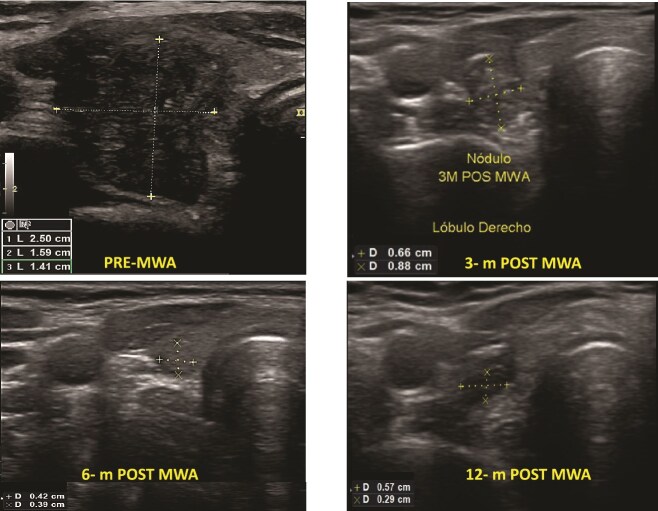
Benign thyroid nodules pre- and post-MWA (3, 6, and 12 months), see the changes in volume and the ultrasound aspect of the nodule.
